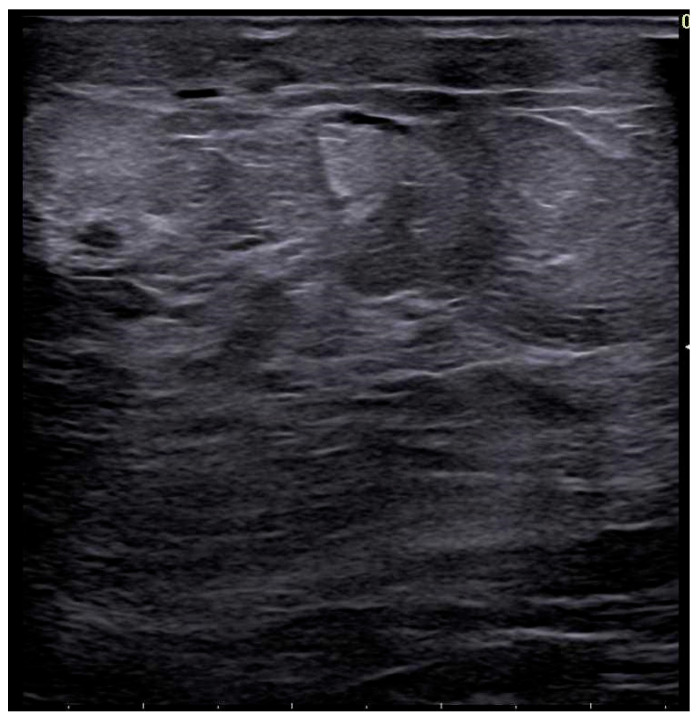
Corresponding ultrasound of post-radiotherapy skin thickening/edema.
